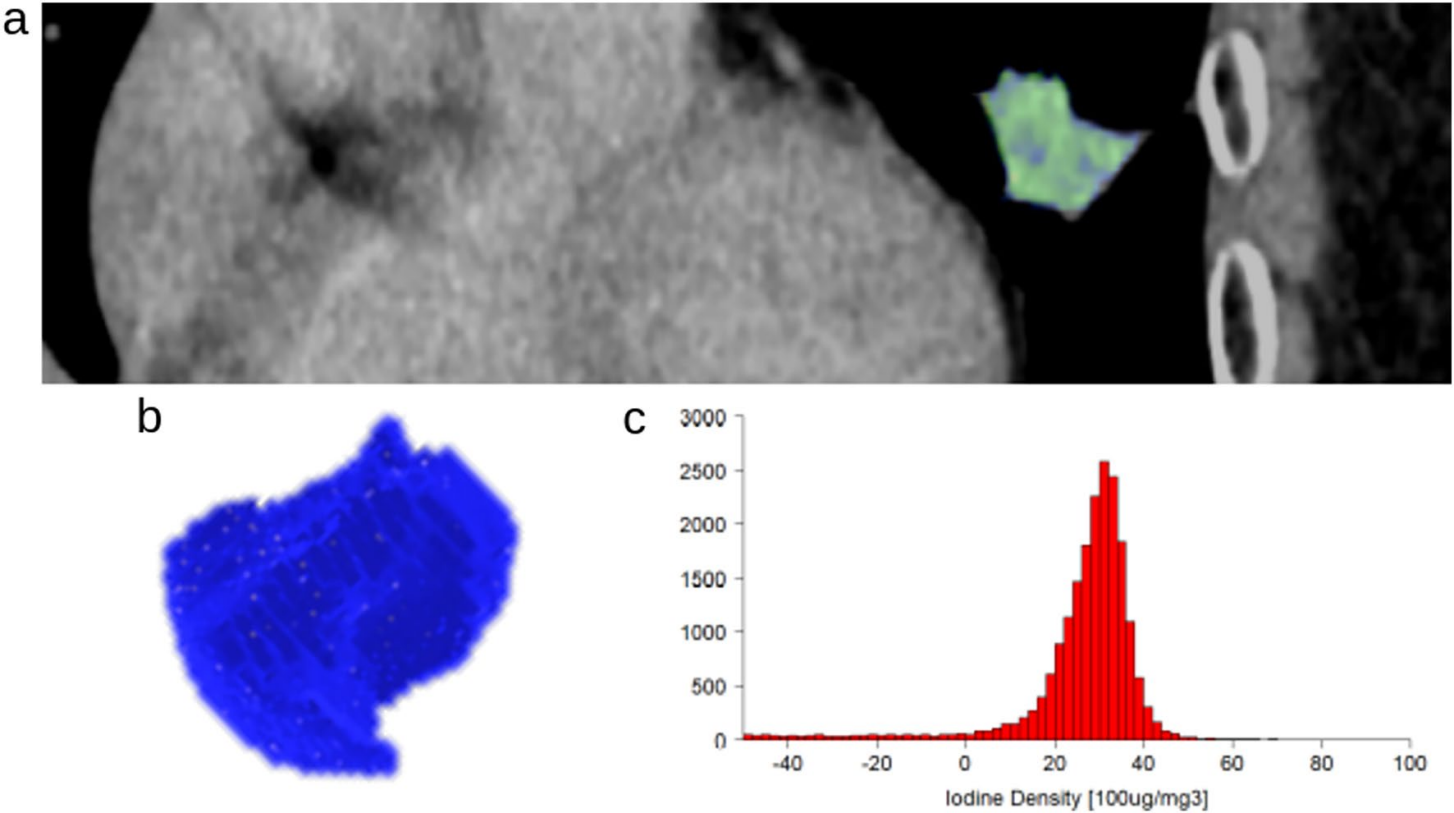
A representative case with PD-L1 positive invasive adenocarcinoma (TPS 80%). A coronal CT image with nodule segmentation (a), 3D volume of interest on tumor (b), and 3D iodine density histogram (c). 3D-iodine density mapping texture features and ECV were as follows: Max, 70 mg/cc; Min, − 108 mg/cc; Median, 29 mg/cc; Average, 20.5 mg/cc; Standard deviation, 28.82 mg/cc; Skewness, − 2.90 (cutoff value ≤ − 2.28); Kurtosis, 11.01; ECV, 27.60% (cutoff value > 21.23%). *PD-L1* programmed cell death 1- ligand 1, *TPS* tumor proportion score; ECV, extracellular volume.
